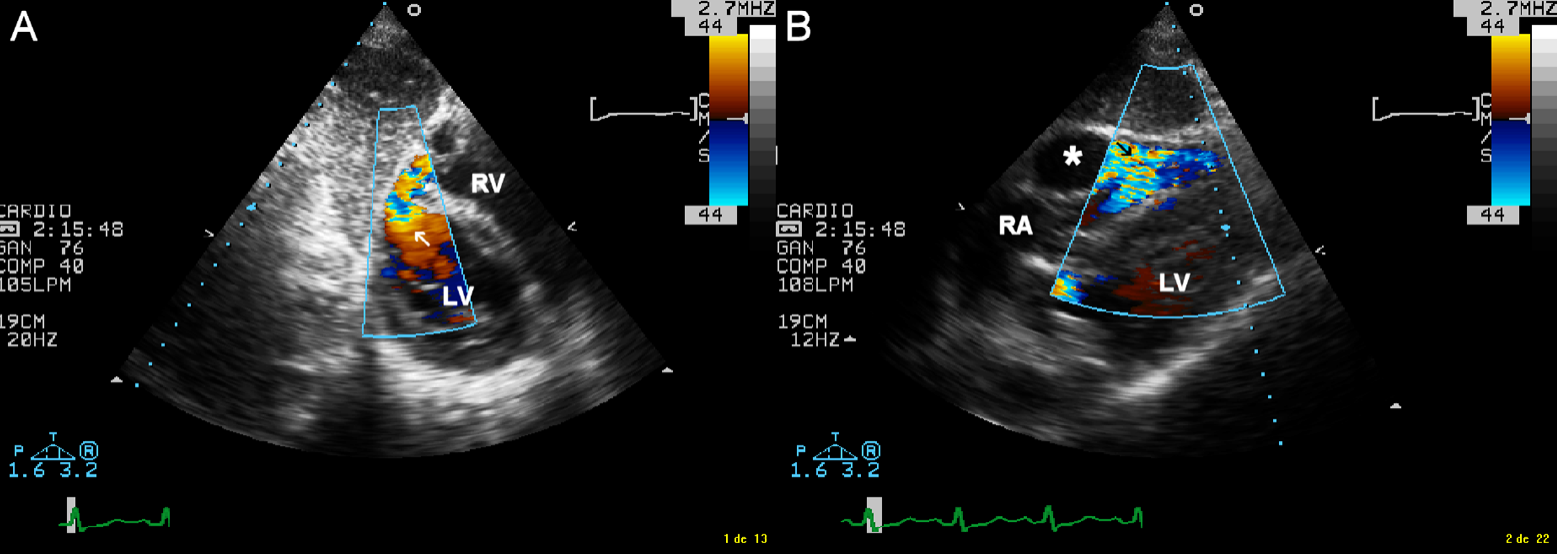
Panel A: transthoracic echocardiography, modified short axis subcostal view with color flow Doppler mapping showing left ventricular entry site of septal rupture (white arrow). Panel B: transthoracic echocardiography, modified four chamber subscostal view with color flow Doppler mapping showing septal rupture exit site in the right ventricular free wall (black arrow). Asterisk indicates the intramural neo-cavity in the right ventricular free wall. Abbreviations: LV, left ventricle, RA, right atrium, RV, right ventricle.
